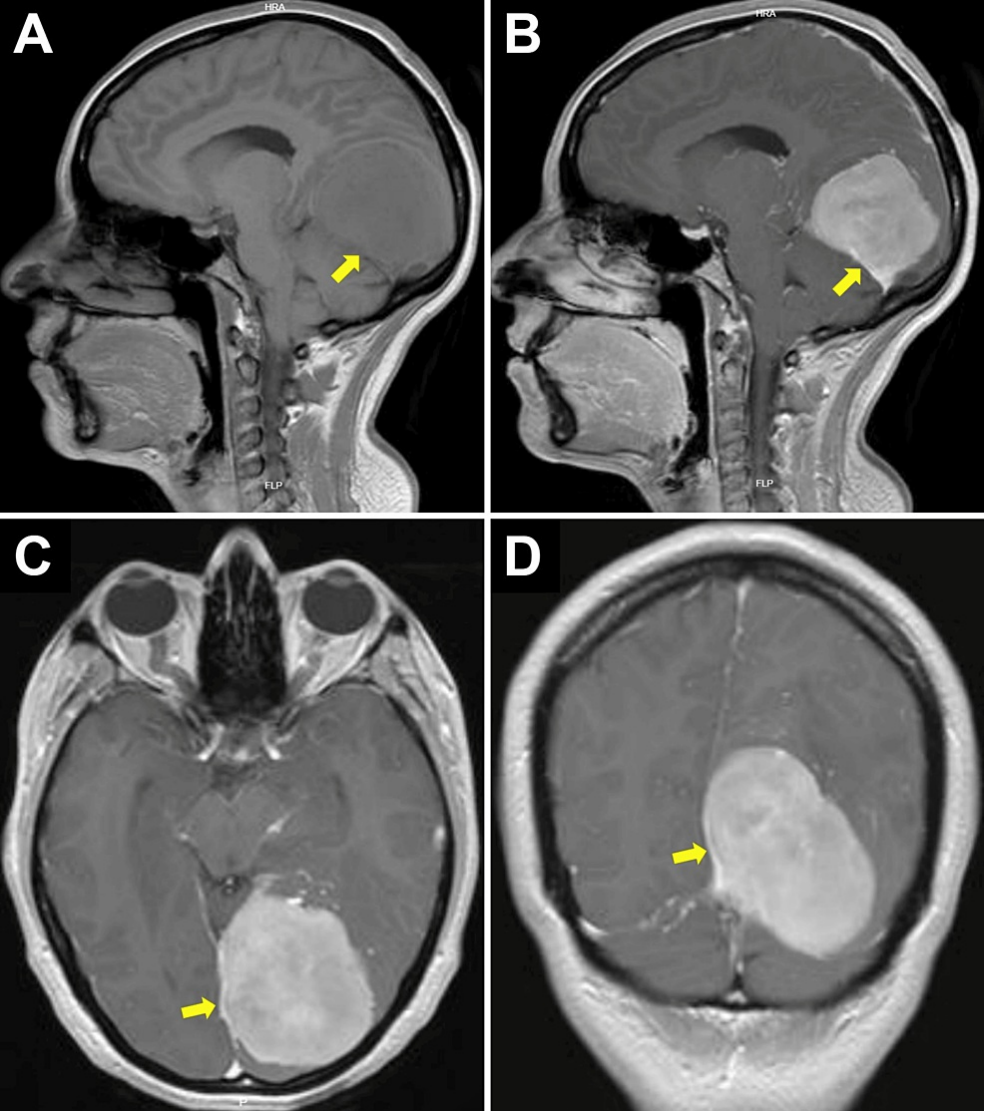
Pre-surgical MRI (Panels A, B) Sagittal MRI views. (A) Sagittal T1-weighted MRI without contrast shows an isointense lesion to gray matter. (B) Contrast-enhanced T1-weighted images show homogeneous enhancement after gadolinium administration. (Panels C, D) Axial and coronal T1-weighted MRI. They show an oval lesion with contrast enhancement of 65 mm maximum diameter, of left supratentorial location in direct relation to the medial aspect of the occipital lobe of the left cerebral hemisphere, with fixation base at the falcotentorial junction along the straight sinus (Fassiouni type IV) and with posterior extension (Bassiouni type IV) (arrows).
